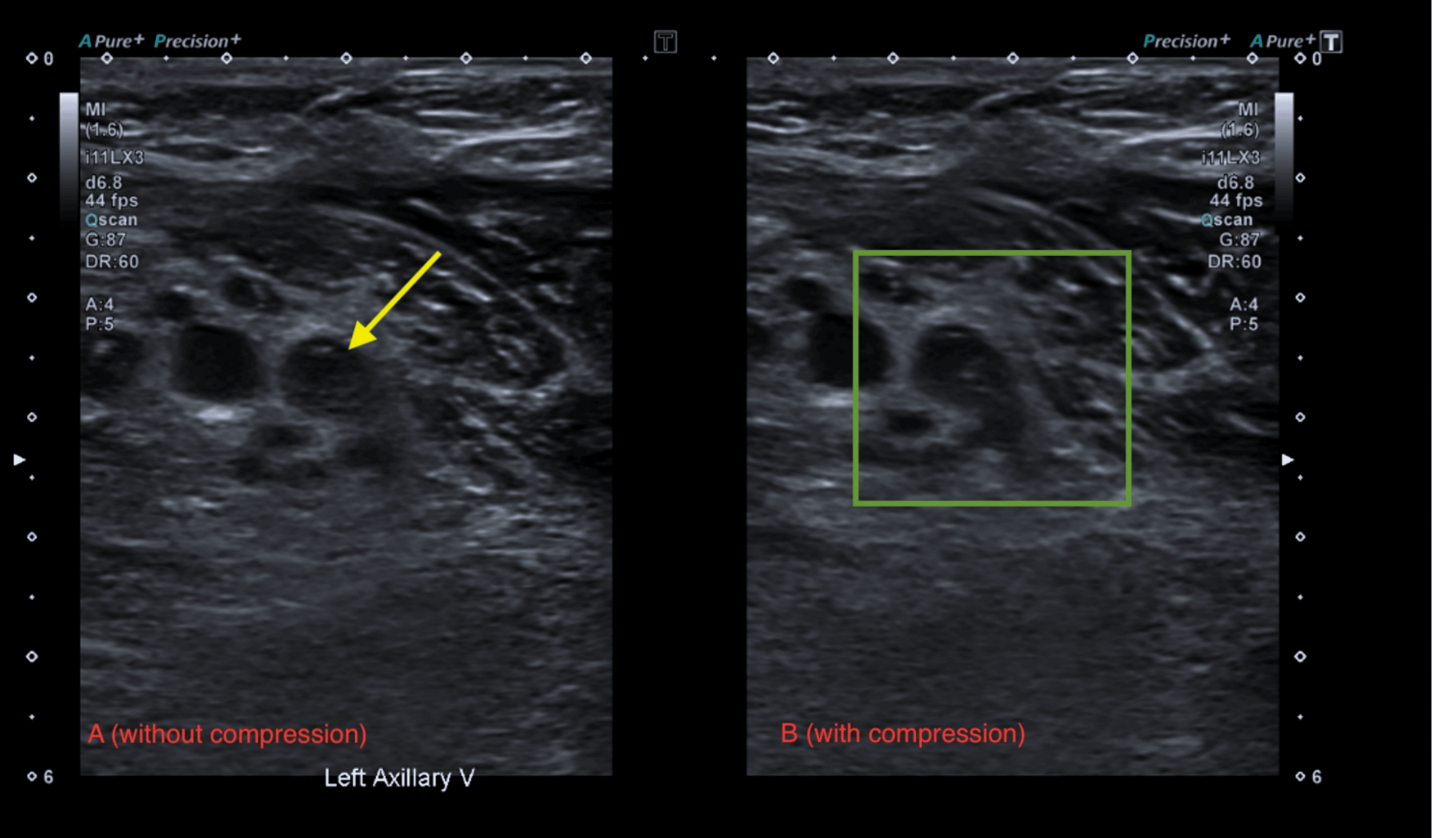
US shows echogenicity (yellow arrow) and lack of compression of the left axillary vein (green box) US: ultrasound (A) US without compression on the left axillary vein. (B) US with compression on the left axillary vein
